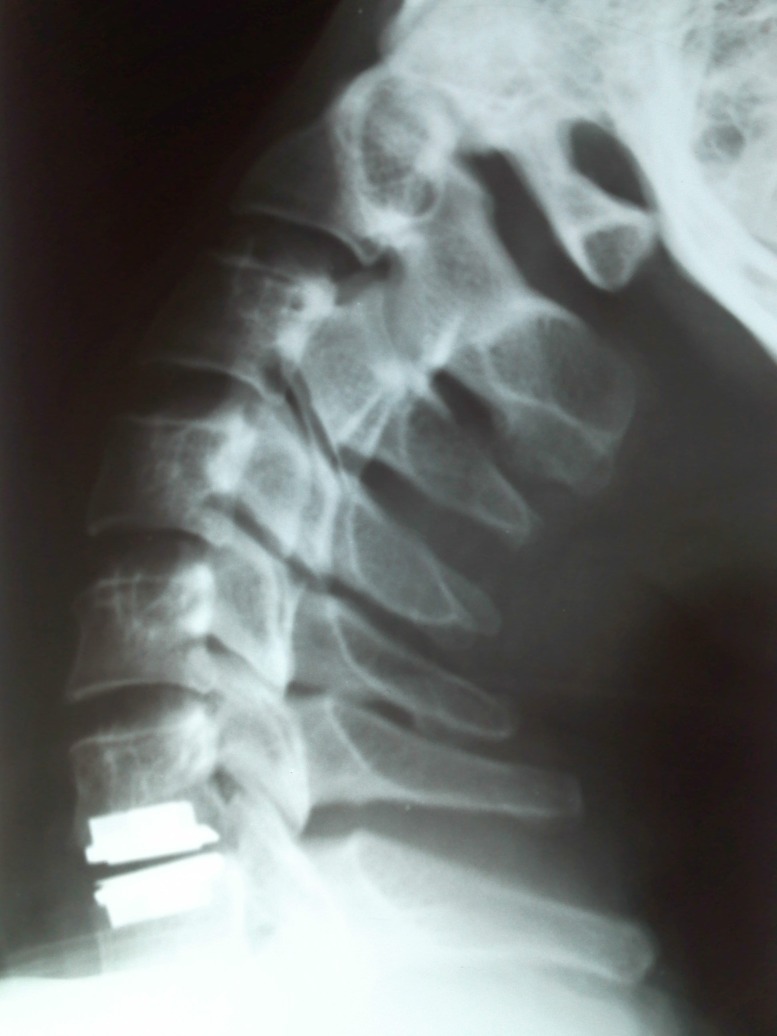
Postoperative cervical x-ray, correct position of Prodisc C prosthesis
